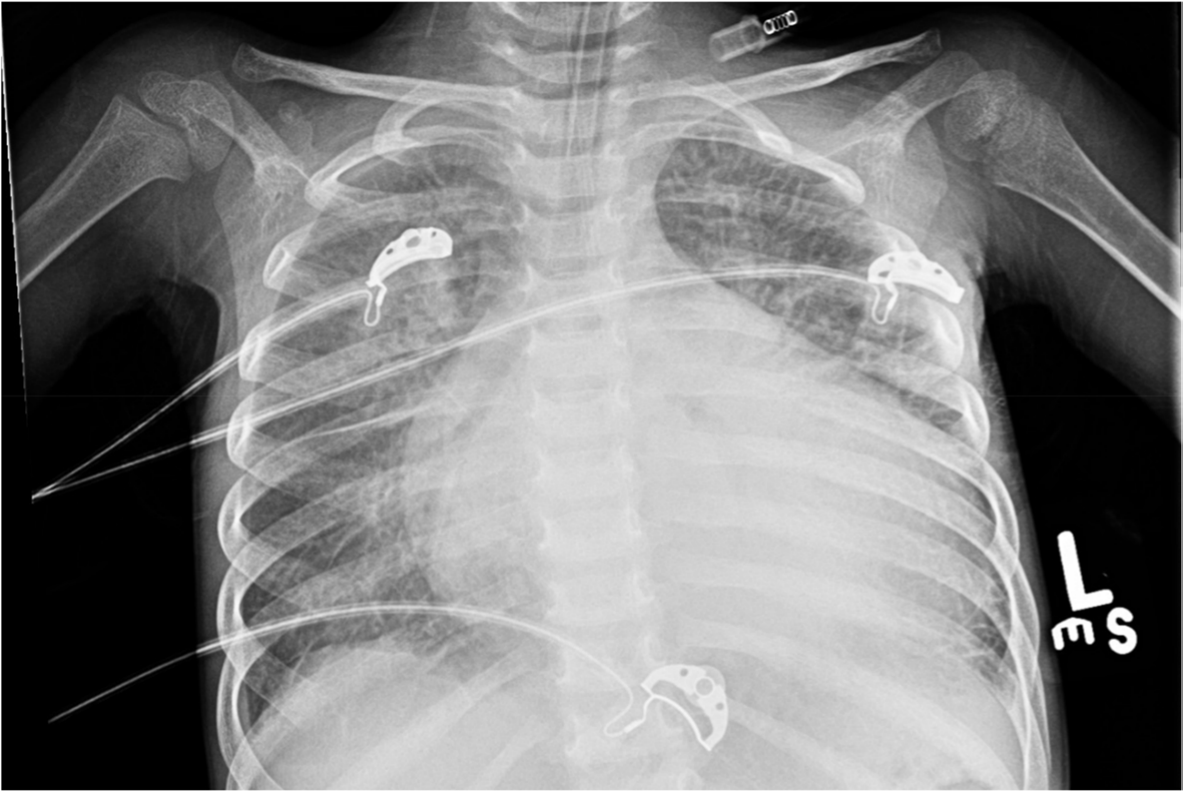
Chest X-Ray upon admission: The cardiac silhouette is significantly enlarged. There are increased interstitial markings with cephalization of the pulmonary vessels and Kerley B lines in keeping with interstitial pulmonary edema. There is blunting of both costophrenic angles suggestive of small bilateral pleural effusions. No pneumothorax. Increased retrocardiac opacity silhouetting the medial aspect of the left hemidiaphragm, atelectatic or infectious in nature
